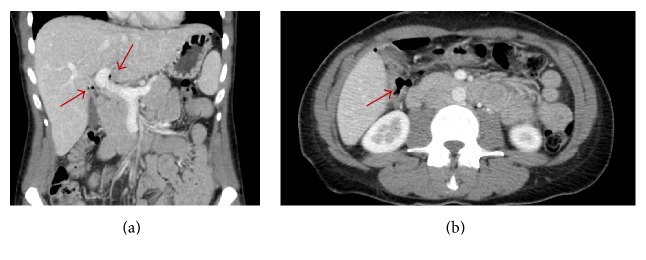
Coronal (a) and transverse (b) views of the CT of the abdomen and pelvis, read as “free intraperitoneal air, findings suggestive of a perforated viscus. There is free air around the porta hepatis which would favor an upper GI source.” Arrows in (a) refer to coronal view of free air around the porta hepatis and in (b) to transverse view of free air around porta hepatis.
